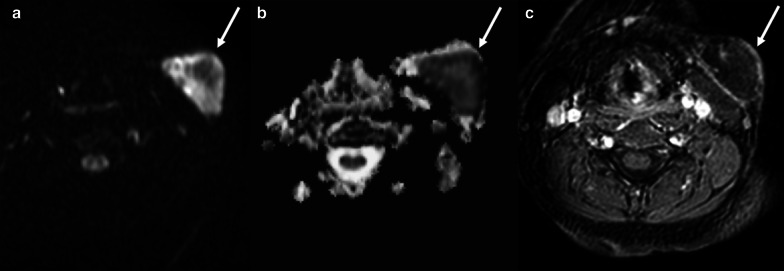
A 62-year-old female patient with pain and swelling in the left side of the neck, fever, and history of larynx cancer treated with RT. Axial high b-value (b = 1000) DWI (a), ADC map (b), and post-contrast gradient-echo fat-suppressed T1-weighted (c) images show an enlarged left laterocervical lymph node (arrows), with very low ADC values (b) and no contrast enhancement (c) due to the purulent content of suppurative lymphadenitis
